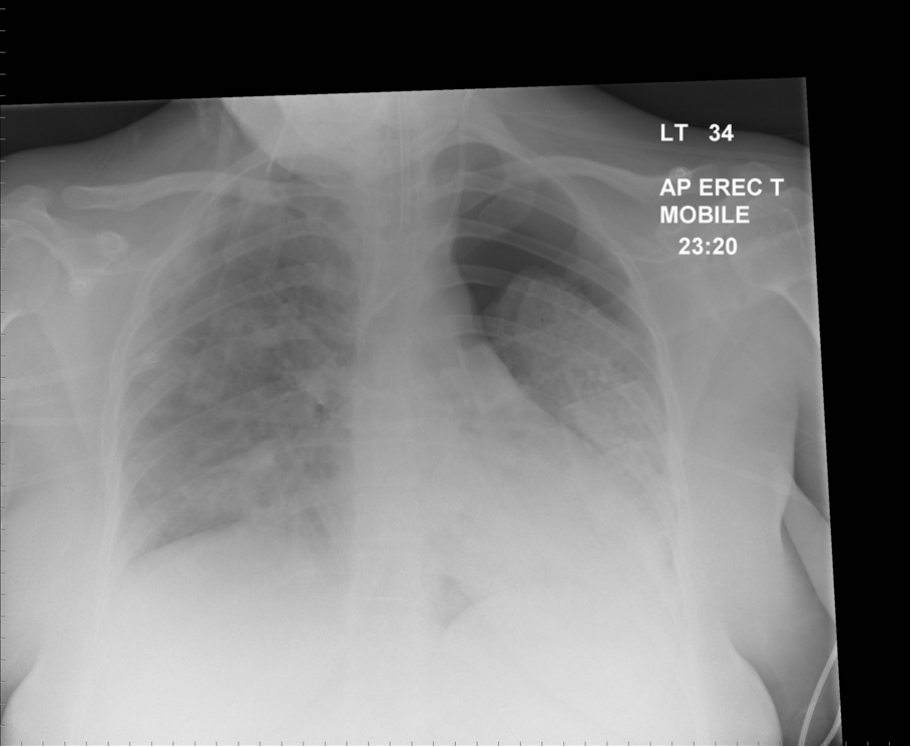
Chest radiograph of our first patient demonstrating a pneumothorax.
